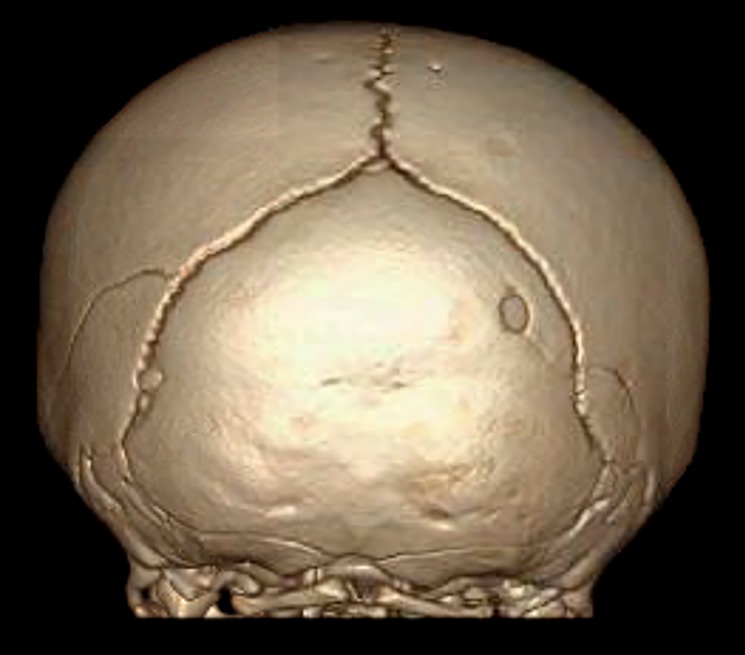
Computed tomography 3-D reconstruction showing bilateral parietal skull fractures including additional lucency on the right that crosses the lambdoid suture and communicates with a small occipital bone defect.
